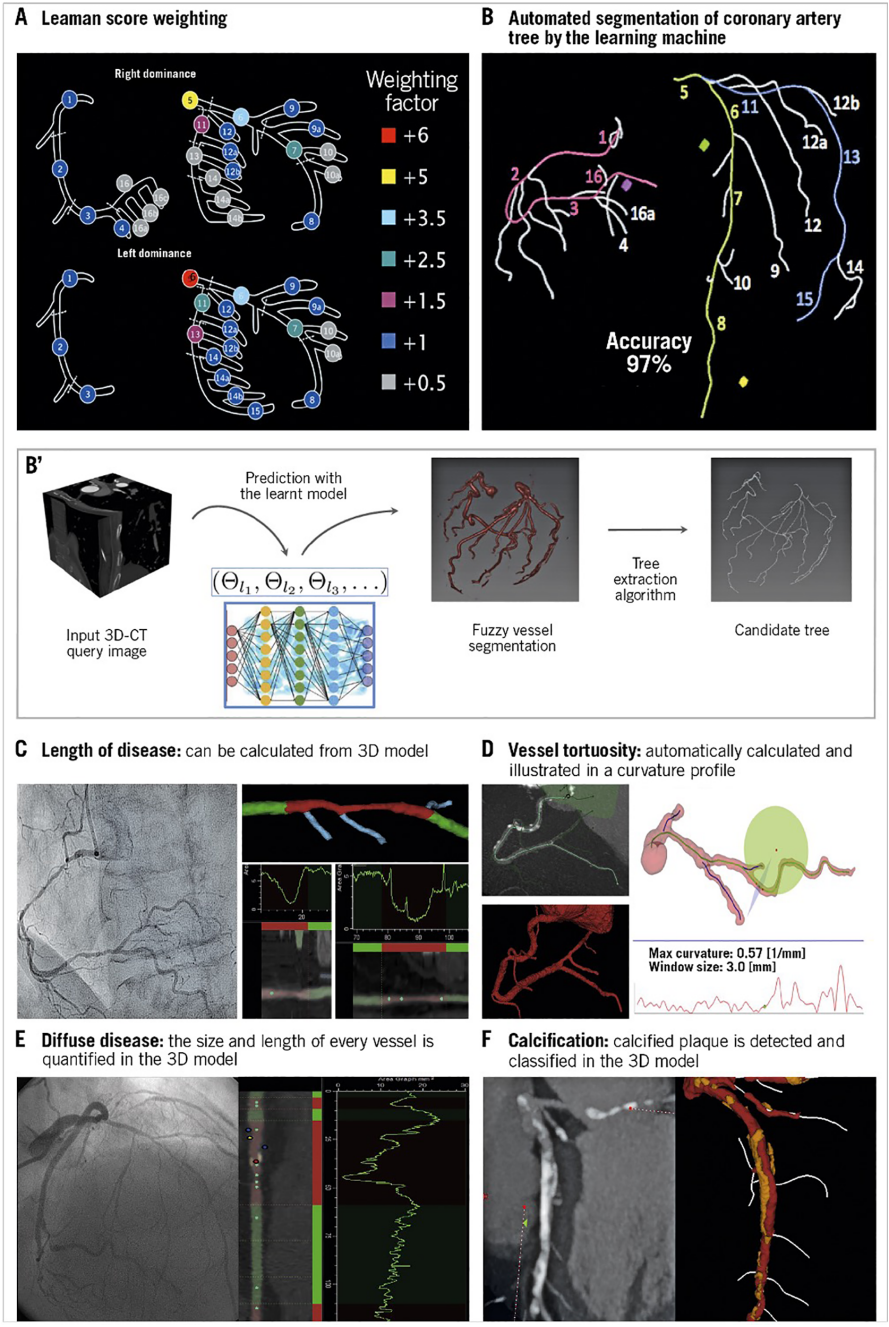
Anatomic SYNTAX score evaluated from the multislice CT scan with automated segmentation produced by the learning machine: (A) Leaman score weighting, (B,B') automated segmentation of coronary artery tree by machine learning, (C) calculating the length of the lesion, (D) estimation of vessel tortuosity, (E) estimation of diffuse disease, and (F) estimation of calcification [Reproduced from Serruys et al. (76)].
